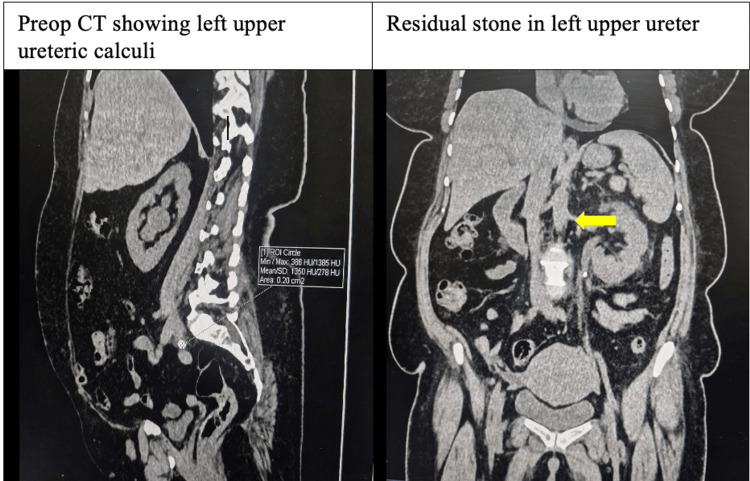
High-density upper ureteric calculus. Preoperative non-contrast computed tomography (NCCT) image demonstrating a high-density upper ureteric calculus with a region of interest (ROI) measurement (mean Hounsfield unit (HU) value, 1,350; maximum HU value, 1,385; area, 0.20 cm²). This patient had a residual stone fragment at the one-month follow-up.
